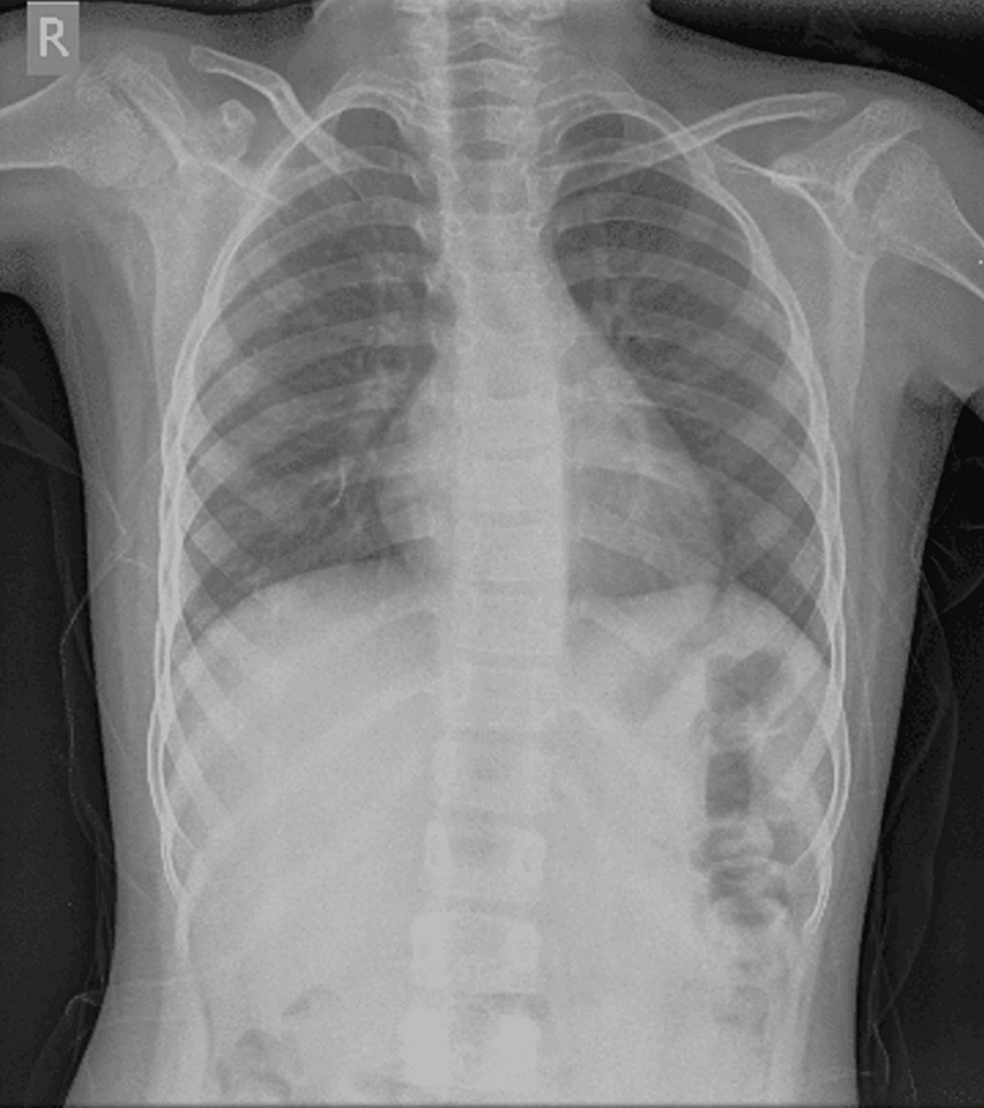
Chest radiograph - posteroanterior view
